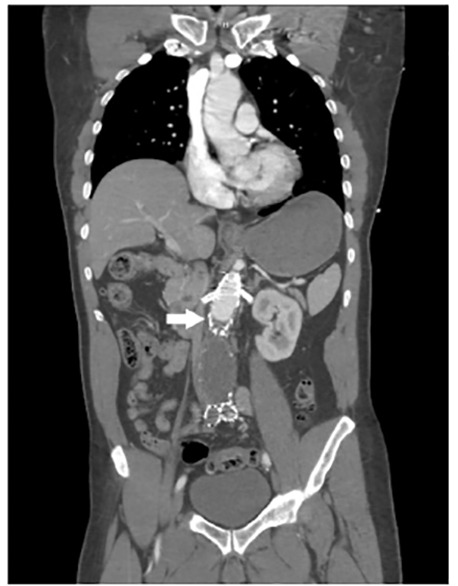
Coronal view of abdominal computed tomography angiography demonstrating contrast reaching but not flowing past the site of occlusion at the aortic endograft (arrow).
